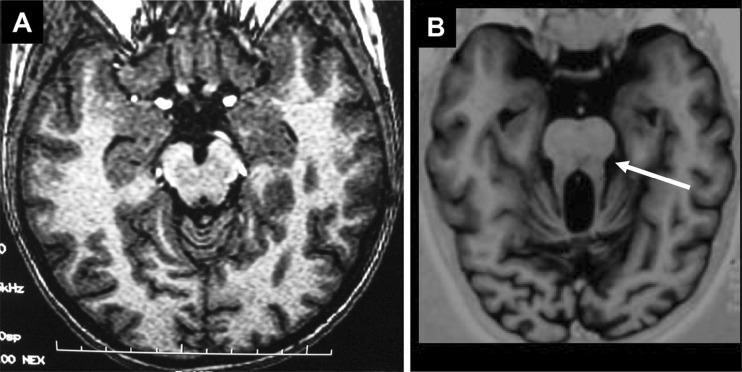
Molar tooth sign on brain magnetic resonance imaging (MRI). Brain MRI axial image at the level of the superior cerebellar peduncles of a control subject (a) and an affected individual (b) showing abnormally increased depth of the interpeduncular fossa, narrowing of the midbrain tegmentum, and thickening of the superior cerebellar peduncles, all of which contribute to the radiologic feature known as the molar tooth sign (*white arrow*)
